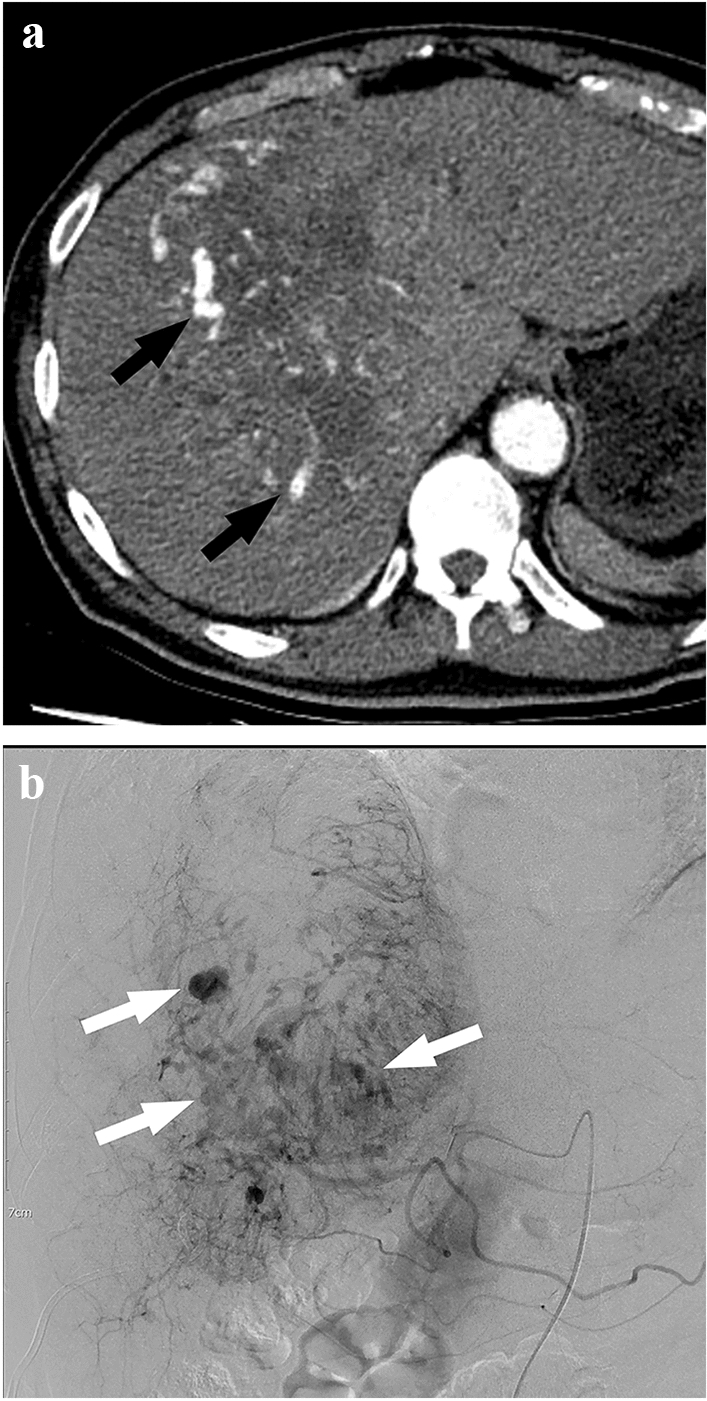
A 67 year old man with hepatocellular carcinoma (size equal to 16 cm). (a,b) Arterial phase CT images (a) and hepatic arteriography (b) revealed multifocal dysmorphic intratumoral vessels with sizes up to 9 mm (arrow). The lung-shunt fraction was 44.74%.
